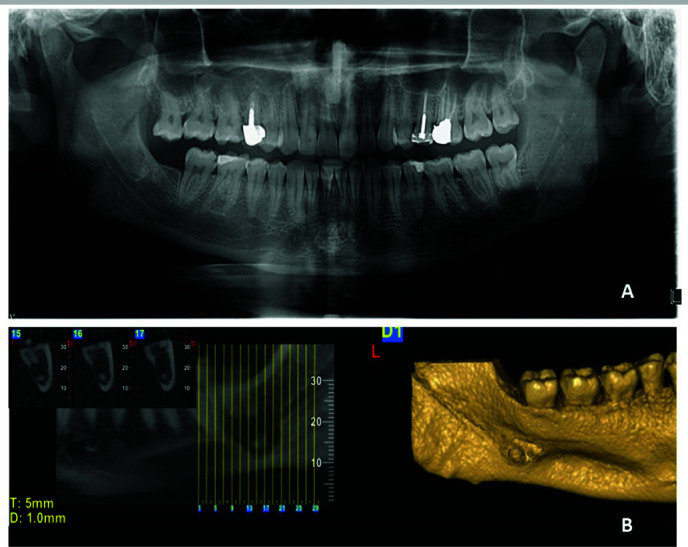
a: Panoramic view of patient with a well-defined mixed radiolucent-radiopaque lesion next to the apex of left mandibular third molar, b: CBCT showed expansion
